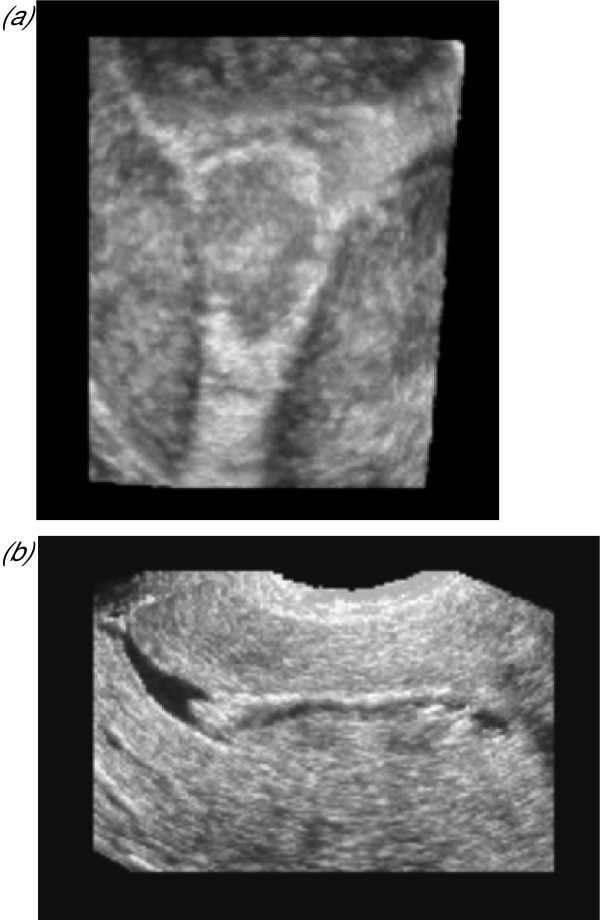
Three-dimensional saline infusion contrast sonography*. Two-dimensional ultrasound had shown a persistently thick endometrium despite several weeks of pituitary suppression prior IVF treatment and saline infusion sonography has proven unhelpful simply confirming a thick endometrium despite very low serum oestradiol levels. A three-dimensional acquisition was undertaken therefore both before (Fig. 9a) and during the instillation of saline (Fig. 9b) and subsequently analysed off-line to reduce the amount of saline used and overall time needed for the examination. A large sub-mucosal leiomyoma with a broad base was seen originating from the left posterior aspect of the uterus that almost entirely occupied the cavity (Fig. 9b). This allowed the cancellation of the planned IVF treatment with the patient continuing her 'down-regulation' medication for a further two-months at which stage the fibroid was removed transcervically using a modified operative hysteroscope.
